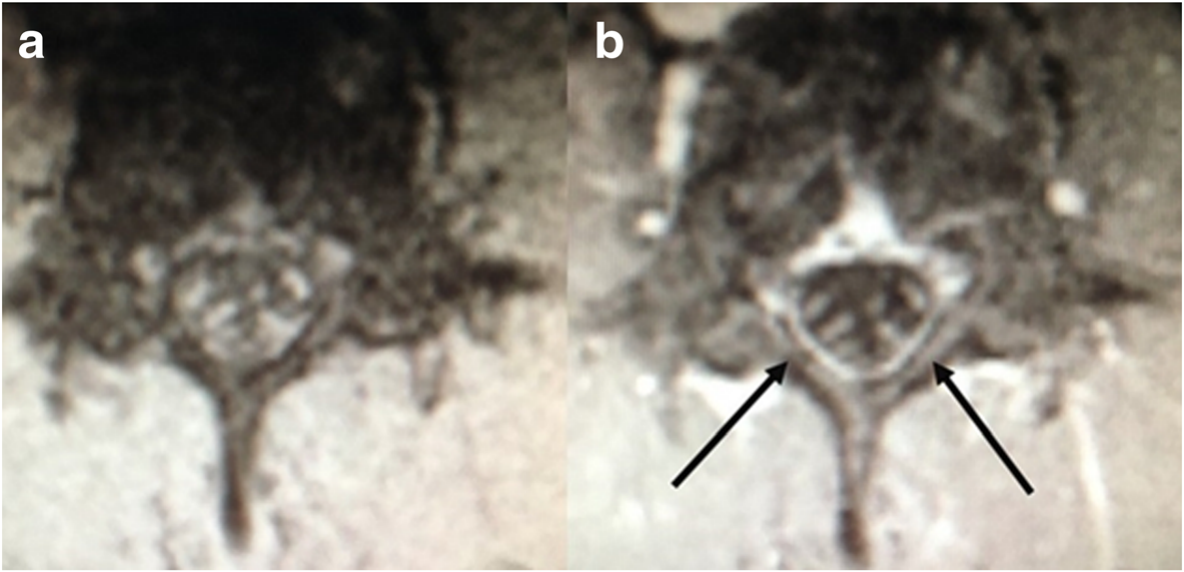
MRI. Transverse section of the L4 vertebral area. a Unenhanced fat-suppressed T1-weighted image. b Gd-contrast enhanced fat-suppressed T1-weighted image. Arrow indicates contrast effects observed in the back of the epidural space
